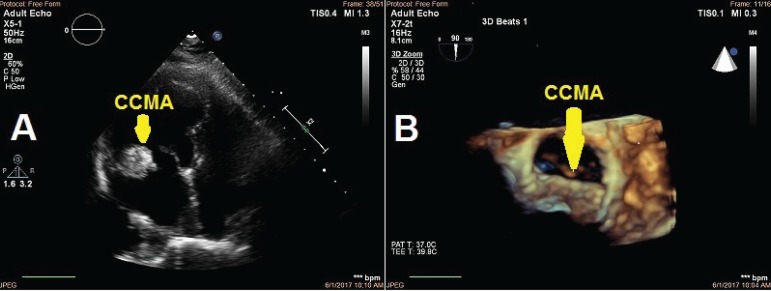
A) Two-dimensional transthoracic echocardiography (in the apical four-chamber view) of a calcified caseous mass attached to the mitral annulus (arrow). B) Three-dimensional transesophageal echocardiography of an irregular surface of CCMA (arrow).
